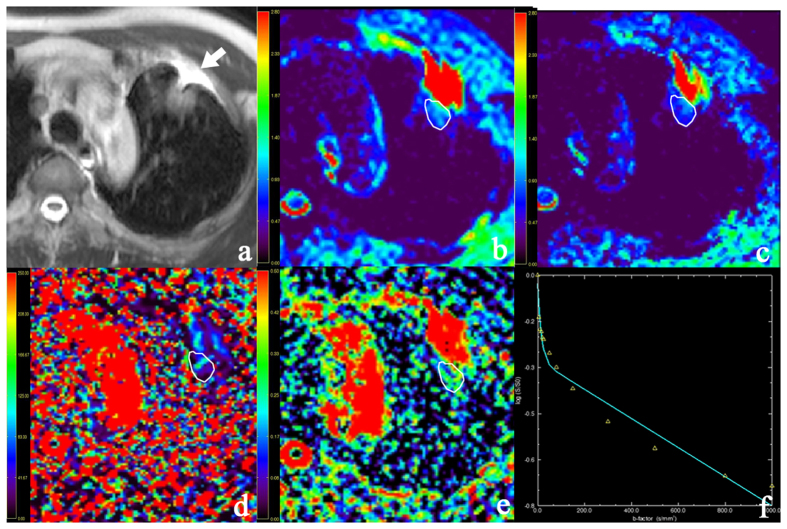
Pleural retraction in a 52-year-old man with left upper lobe adenocarcinoma. (a) T2-weighted image. (b) Apparent diffusion coefficient (ADC) map. (c) The D map (d) The D* map. (e) The f map. (f) The bi-exponential fitting of the diffusion signal decay. Pleural retraction (arrow) demonstrates triangle-like hyper-intensity on T2WI. It possesses high ADC, D, f values and low D* values. The tumor (partly showed) show impeded diffusion on ADC and D maps (encoded in blue color).
